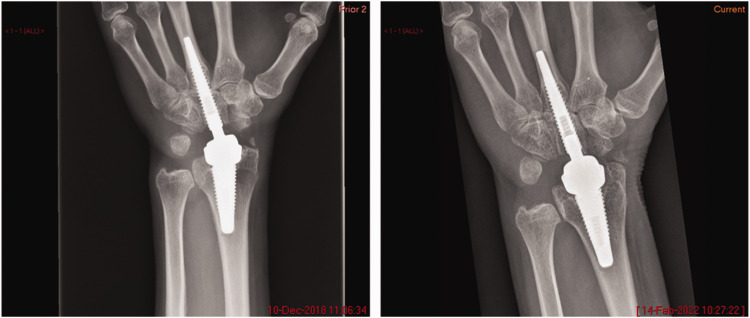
Radiographs of a well-fixed metacarpal stem of Motec 4 (left) and a later radiograph showing osteolysis and implant subsidence (right).
